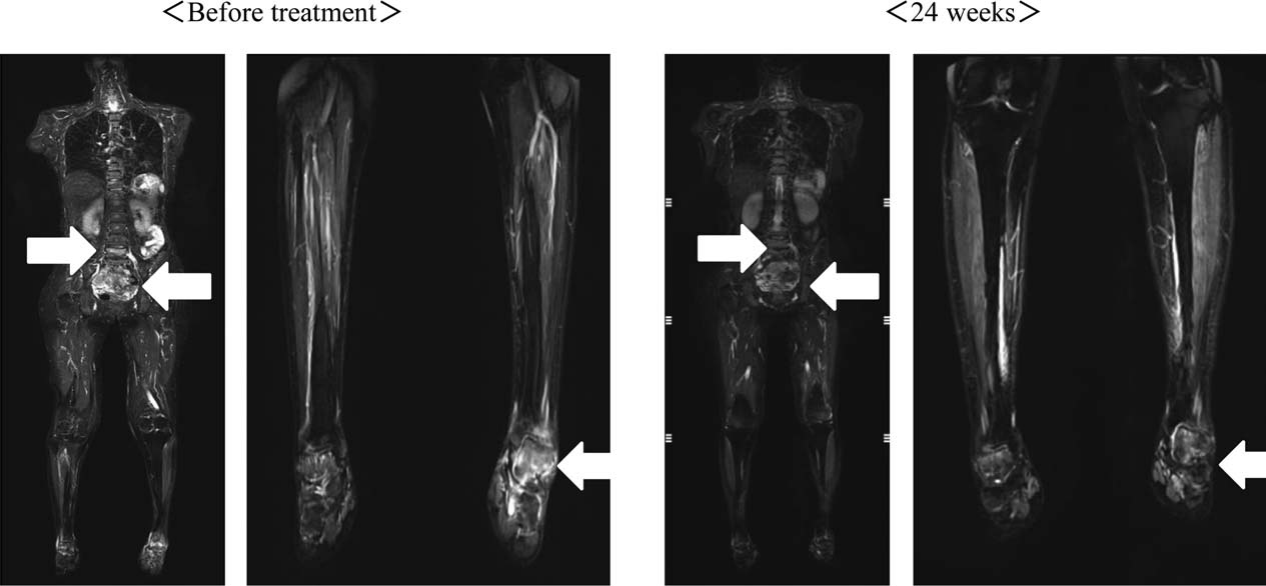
Short-tau inversion-recovery sequence magnetic resonance imaging of the whole body and lower legs. High signal intensity was observed in the talar, sacral, and L5 vertebral regions, indicating multiple pseudofractures that improved after treatment for 24 weeks (white arrows).
